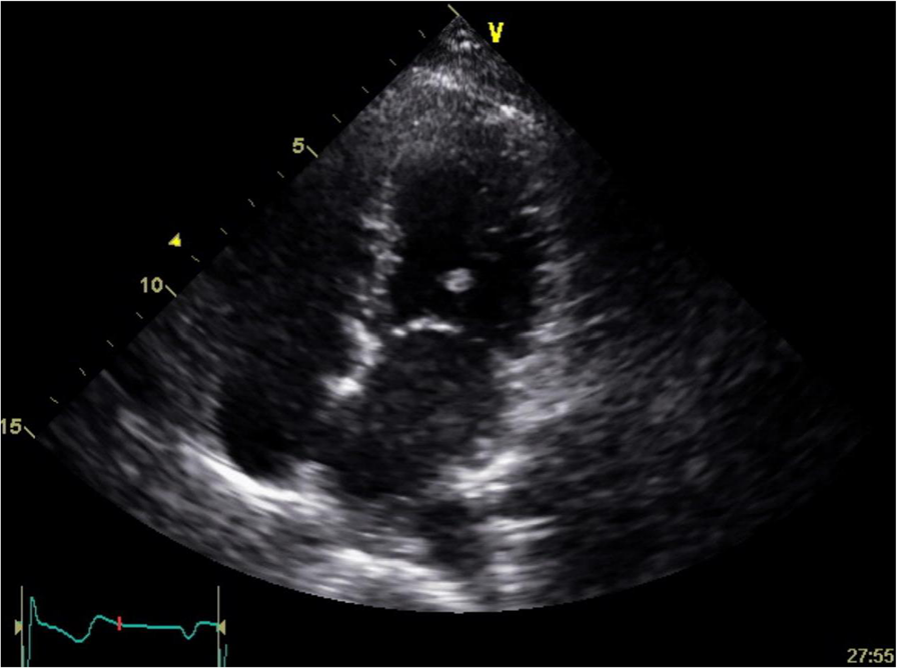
Transthoracic echocardiography showing a tumor (5 × 6 mm) on the chordae of anterior leaflet of mitral valve.
